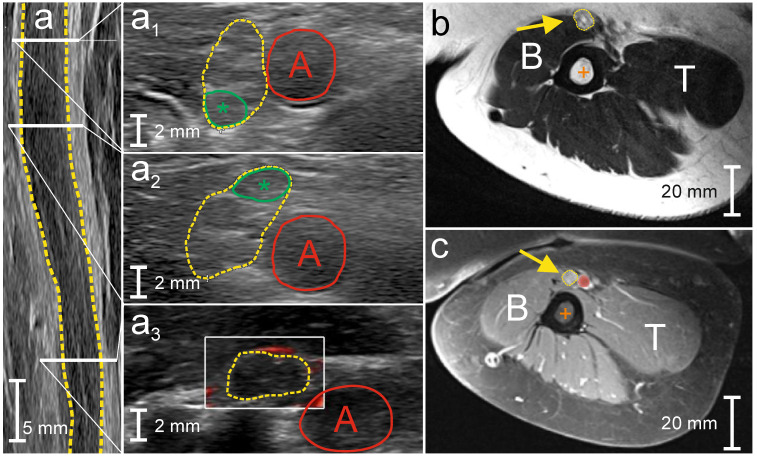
Peripheral nerve imaging in patient 2 (MADSAM). High-resolution nerve ultrasound (HRUS) demonstrated severe, asymmetric, fusiform and segmental enlargement of the cross-sectional nerve area (CSA, yellow in (a–a_3_)) of the left upper arm median nerve ((a_1_) CSA 23.7 mm^2^; (a_2_) 32.1 mm^2^; (a_3_) 12 mm^2^) and isolated fascicle area enlargement (green asterisk in (a_1_) and (a_2_), area in (a_1_) 5.6 mm^2^; in (a_2_) 6.1 mm^2^). Nerve vascularization was not increased (grade 0; within the yellow border in (a_3_)) 3T magnetic resonance neurography (MRN) of the left upper arm median nerve displayed isolated fascicles with contrast-enhancement (yellow arrow in (b) contrast-enhanced (gadolinium) T1-weighted sequence) and T2 hyperintensity (yellow arrow in (c) T2-weighted turbo spin echo sequence with fat saturation). Red dot, brachial artery; A, brachial artery; orange cross, humerus; B, biceps brachii muscle; T, triceps brachii muscle. In a, the long-axis HRUS of the left upper arm median nerve view is given, while (a_1_–a_3_) show the corresponding short-axis view.
